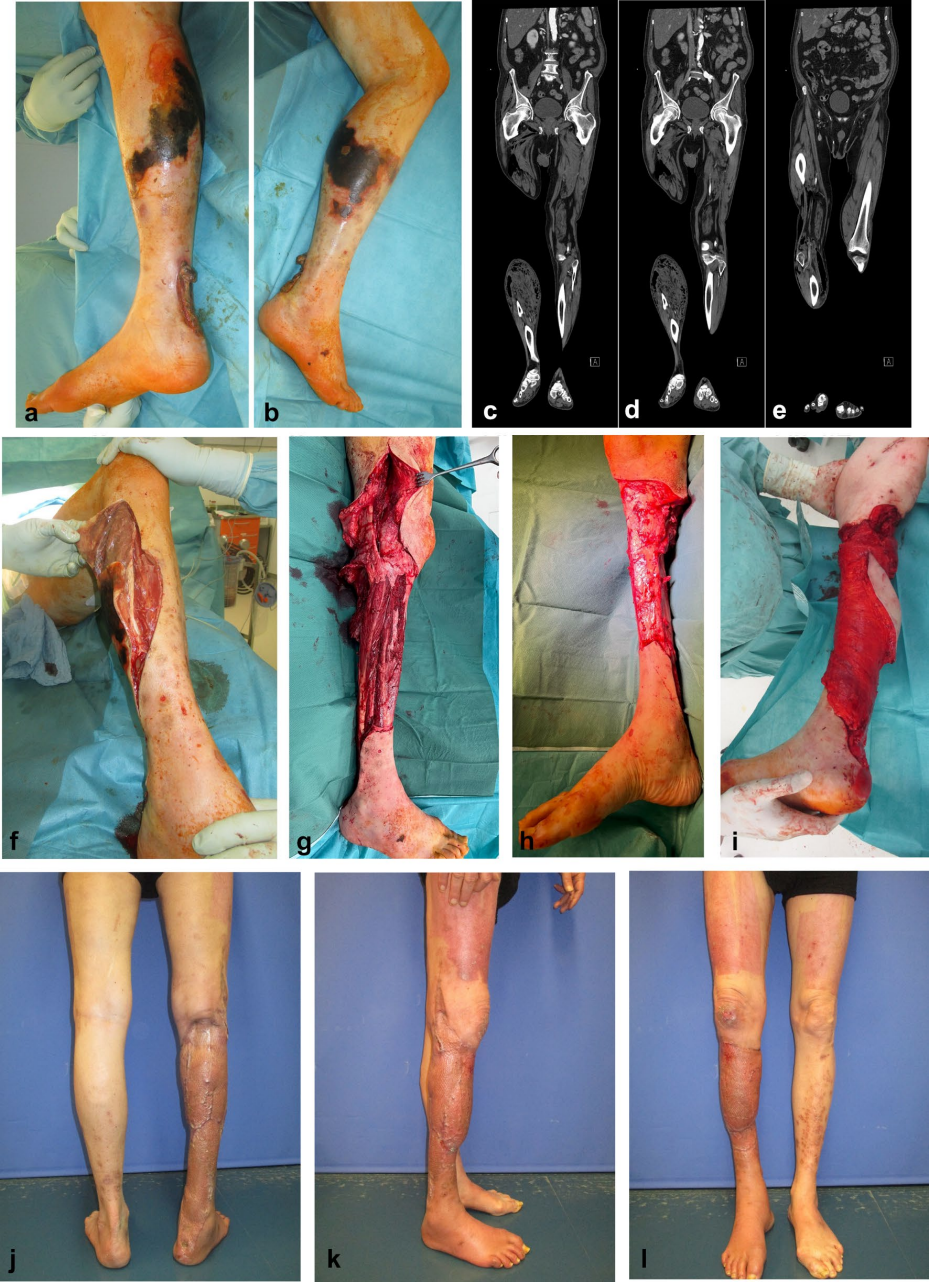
Case report 2: 63-year-old male with necrotizing fasciitis on top of a neglected AT wound after a minor injury. a Initial clinical finding, medial view. b Initial clinical finding, lateral view. c, d, e CT scan depicting air pockets from the leg up to the thigh and gluteal region. f Abscess formation around the muscle fascia of the leg. g Radical debridement; the necrotic muscle fascia was resected together with the complete necrotic triceps surae muscle and AT; finally, the wound was packed in antiseptic bandages; the patient recovered fast and was transferred to a normal ward; empiric antibiotic therapy was initiated and later adapted to the antibiograms. h Follow-up surgery with clean wounds and exposed anterolateral tibia; NPWT was initiated. i Free latissimus dorsi flap transferred for soft tissue coverage. j, k, l Final result 4 months after initial admission
